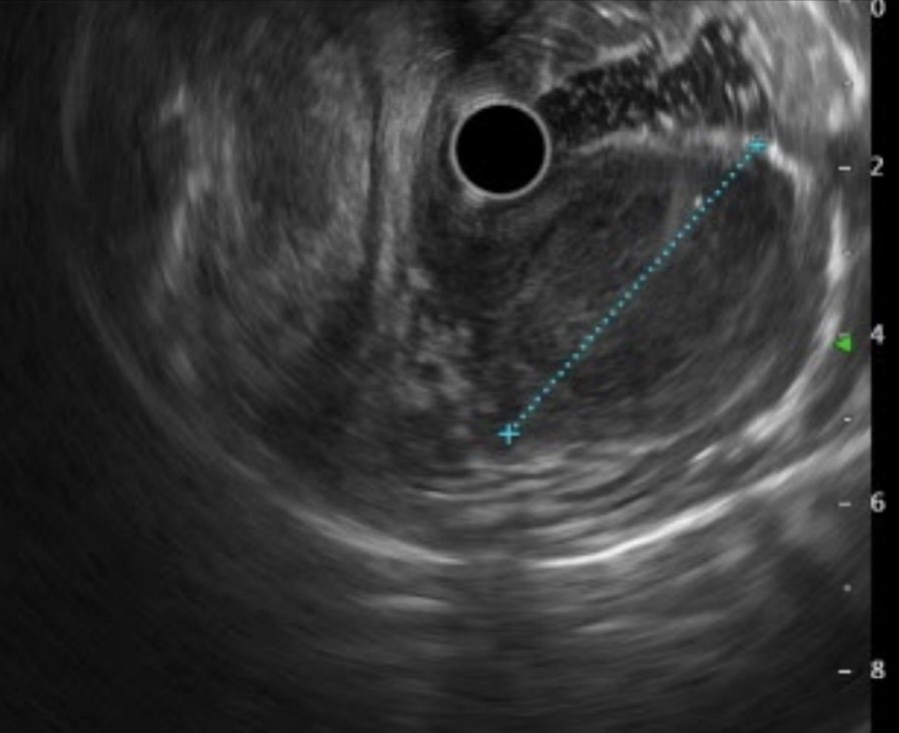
Endoscopic ultrasound revealed a 50-mm oval heteroechoic tumor arising from the muscularis propria (fourth layer) with a slight blood flow signal
